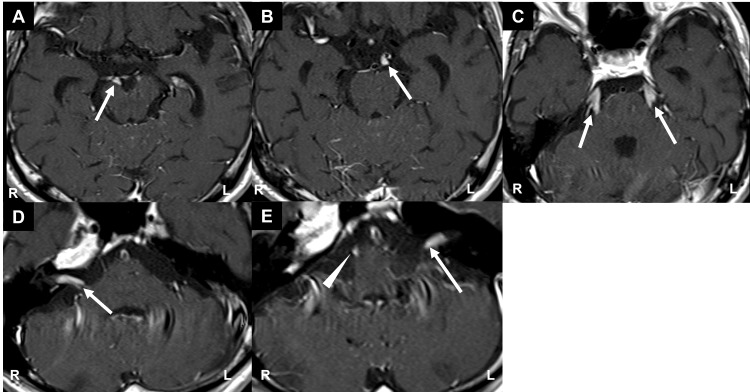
Contrast-enhanced T1-weighted MRI showing enhancement of bilateral oculomotor nerves (A, B; arrows), bilateral trigeminal nerves (C; arrows), bilateral facial nerves (D, E; arrows), and the right hypoglossal nerve (E; arrowhead)
